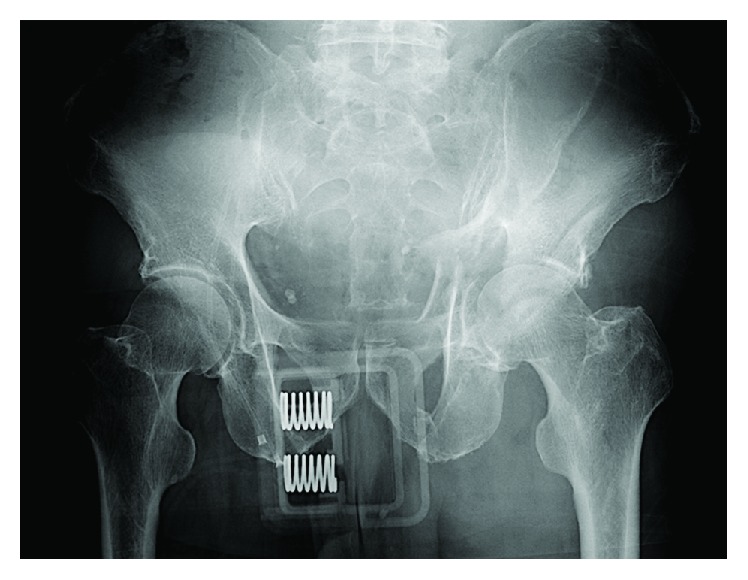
Initial radiographs of the fracture sites. An anteroposterior pelvic radiograph showing the fracture of both columns of the left acetabulum.
